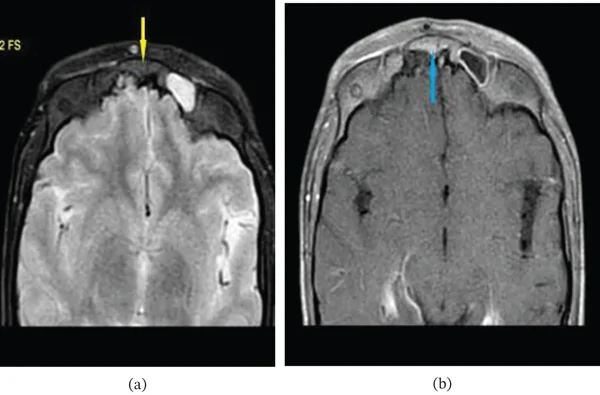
MRI brain with orbits showed T2 Fat Sat hyperintensity (a, yellow arrow) with postcontrast enhancement (b, blue arrow) in the frontal bone with surrounding scalp stranding suggesting osteomyelitis.
